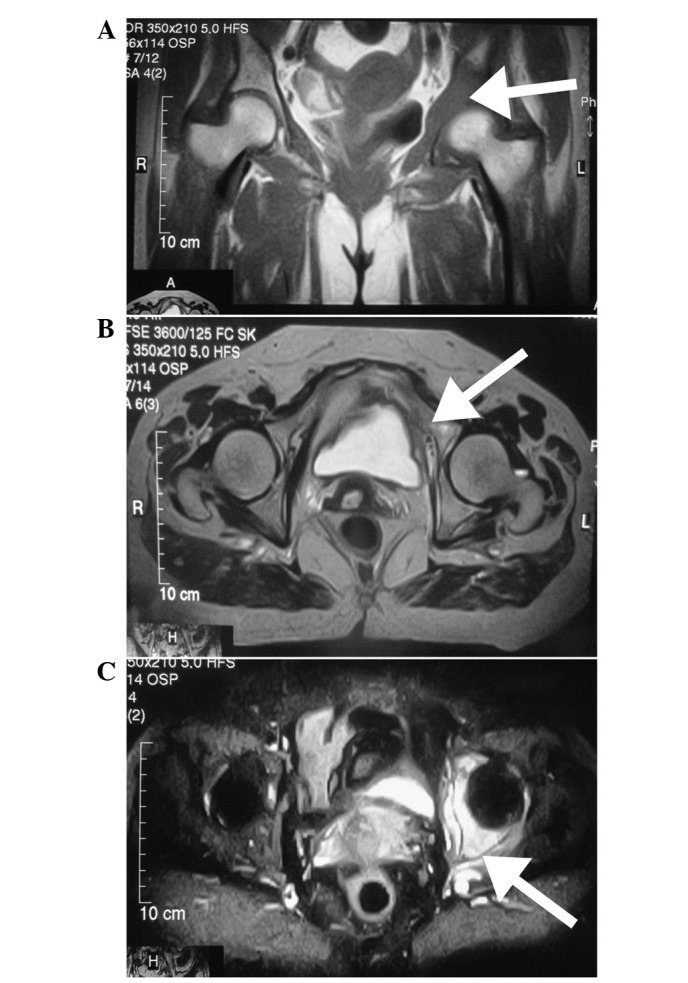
Magnetic resonance imaging showing cortical disruption of the acetabulum and a soft-tissue formation. The signal intensity of the soft-tissue section was (A) mostly homogeneously hypointense (arrow) compared with the bone on T1-weighted images, (B) isointense or slightly hyperintense (arrow) on T2-weighted images and (C) hyperintense (arrow) on short TI inversion recovery.
